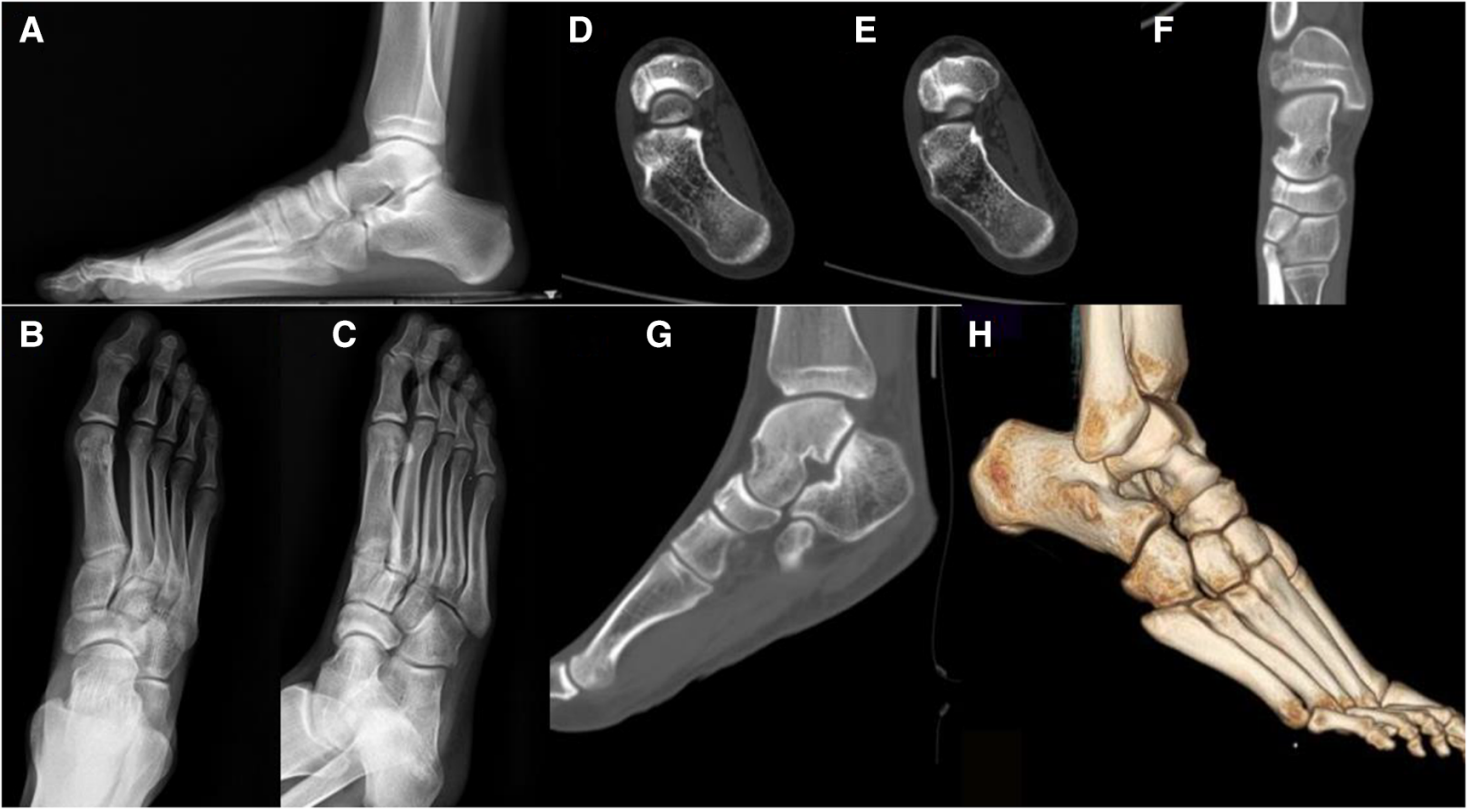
Preoperative imaging data of the right foot showed a tarsal navicular fracture of the right foot (Sangeorzan Type III.2). The fracture line extended from dorsolateral to plantar, and the medial column was stable. (A) Weight-bearing lateral radiographs of the right foot; (B) anteroposterior x-ray of the right foot; (C) oblique radiography of the right foot; (D,E) CT axial view of the right foot; (F) CT coronal view of the right foot; (G) CT sagittal view of the right foot; (H) 3D CT reconstruction of the right foot.
